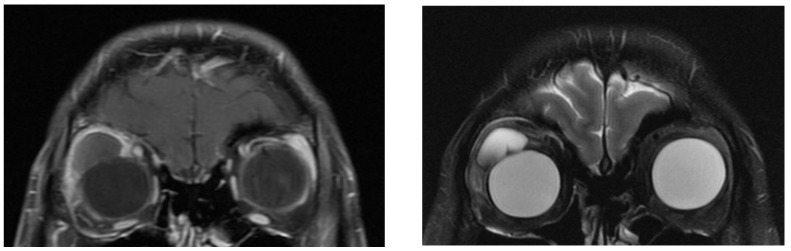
MRI of hydrogel scleral buckles were hypointense in T1 (left) and hyperintense in T2 (right) images.
